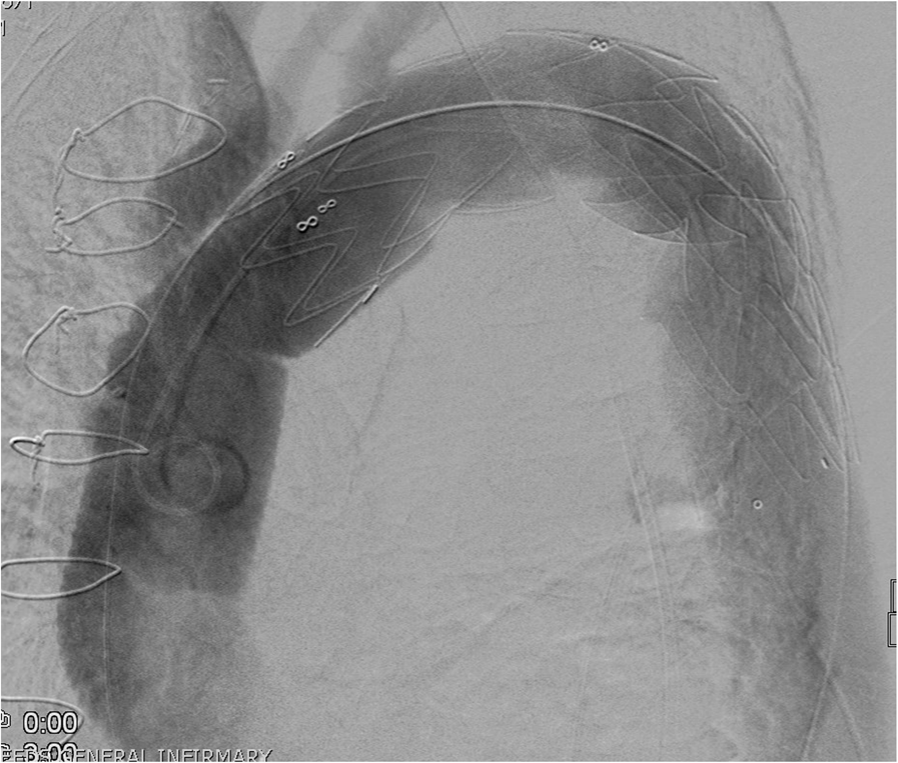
CT scan showing TEVAR of descending thoracic aorta
